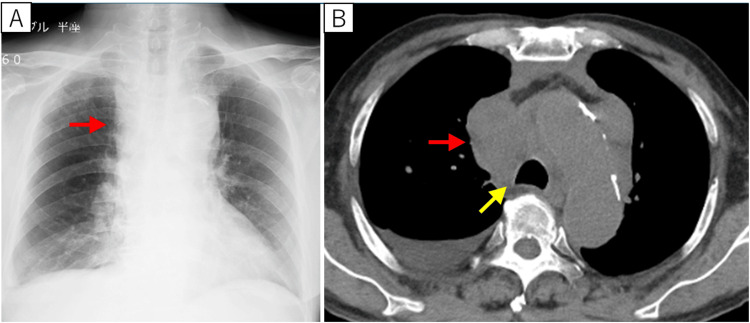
Chest images after palliative radiotherapy to the mediastinum and steroid administration After treatment began, the mediastinal mass lesion was significantly reduced on chest X-ray (A) and chest CT (B) (red arrow), and the compressive narrowing of the trachea improved (yellow arrow) CT: computed tomography
